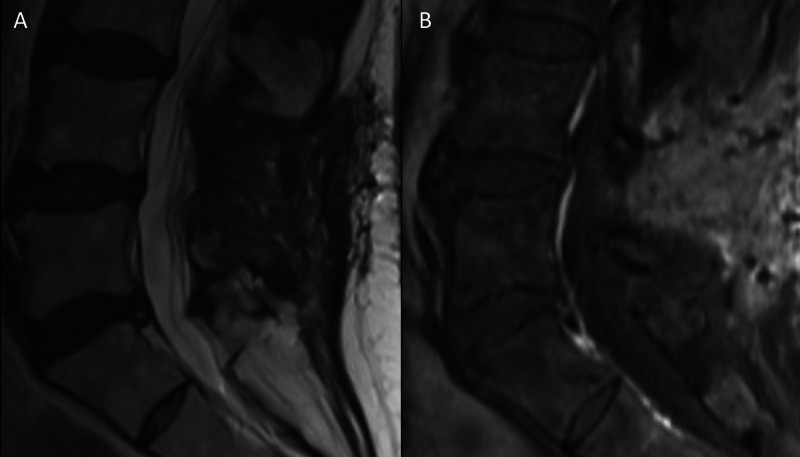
Postoperative MRI showing total resection (A and B) T2 MRI and T1 post-contrast MRI, respectively, showing complete resection and no complication
